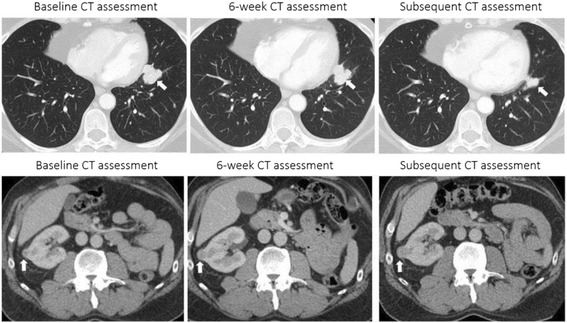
Computed tomography (CT) scans at baseline, 6-week, and subsequent assessment for two separate mRCC patients treated with PD-1/PD-L1 ICB. The first patient (upper panels) had stable disease (SD) on 6-week scan with a 34% decrease in NLR from baseline and subsequently displayed partial response (PR) on next assessment. The second patient (lower panels) had SD on 6-week scan with a 113% increase in NLR from baseline and subsequently displayed progressive disease (PD) on next assessment. Arrows (white) show change in selected area of disease burden
